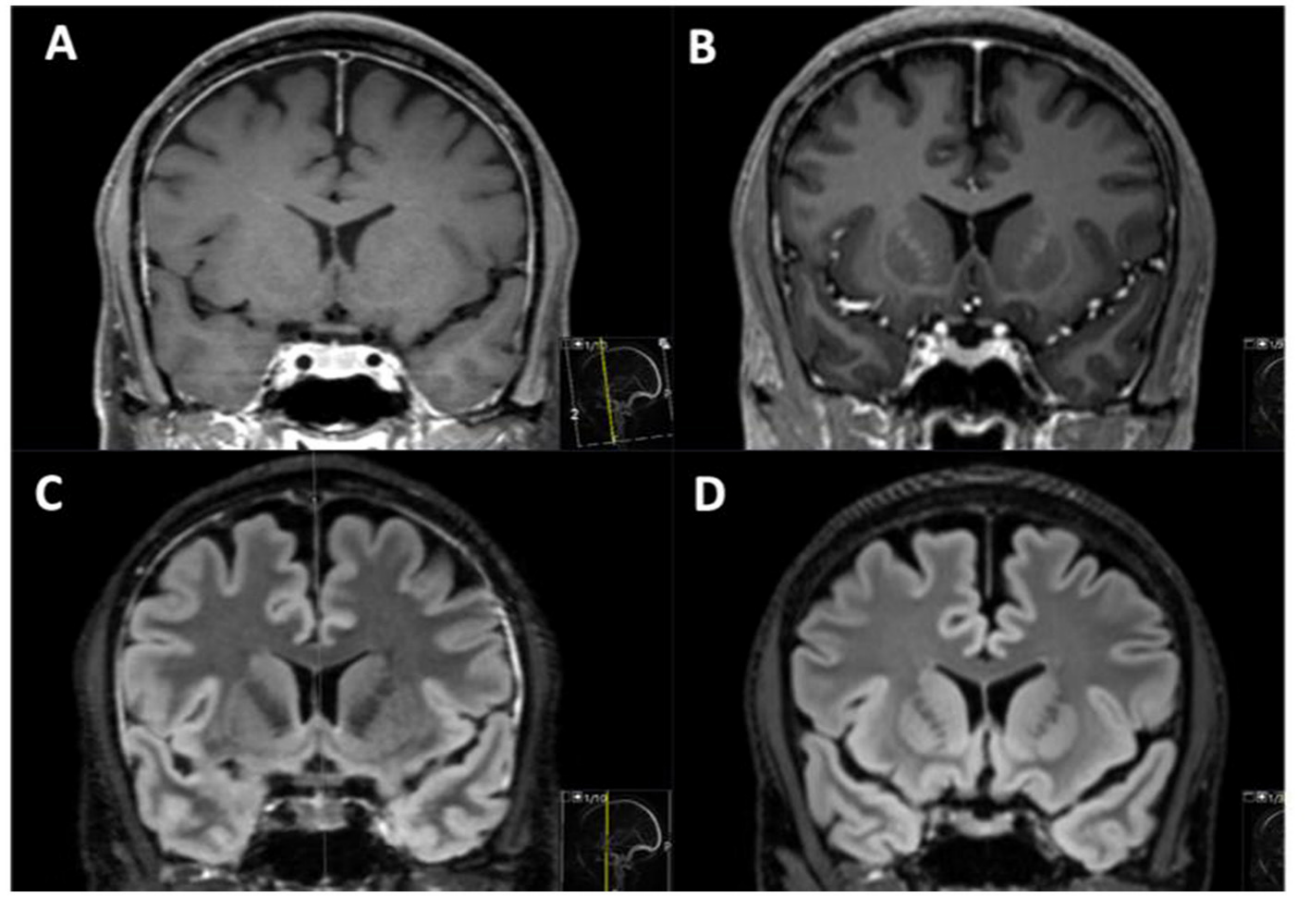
MRI in one patient without venous lake thrombosis investigated before (B, D) and after (A, C) lumbar puncture to investigate headache. After lumbar puncture, thickened dural enhancement can be noted on T1 Gd images (A), and an increased signal on T2 FLAIR (C) in the widened dura can be observed, with an increase in pituitary eight (A, C).
